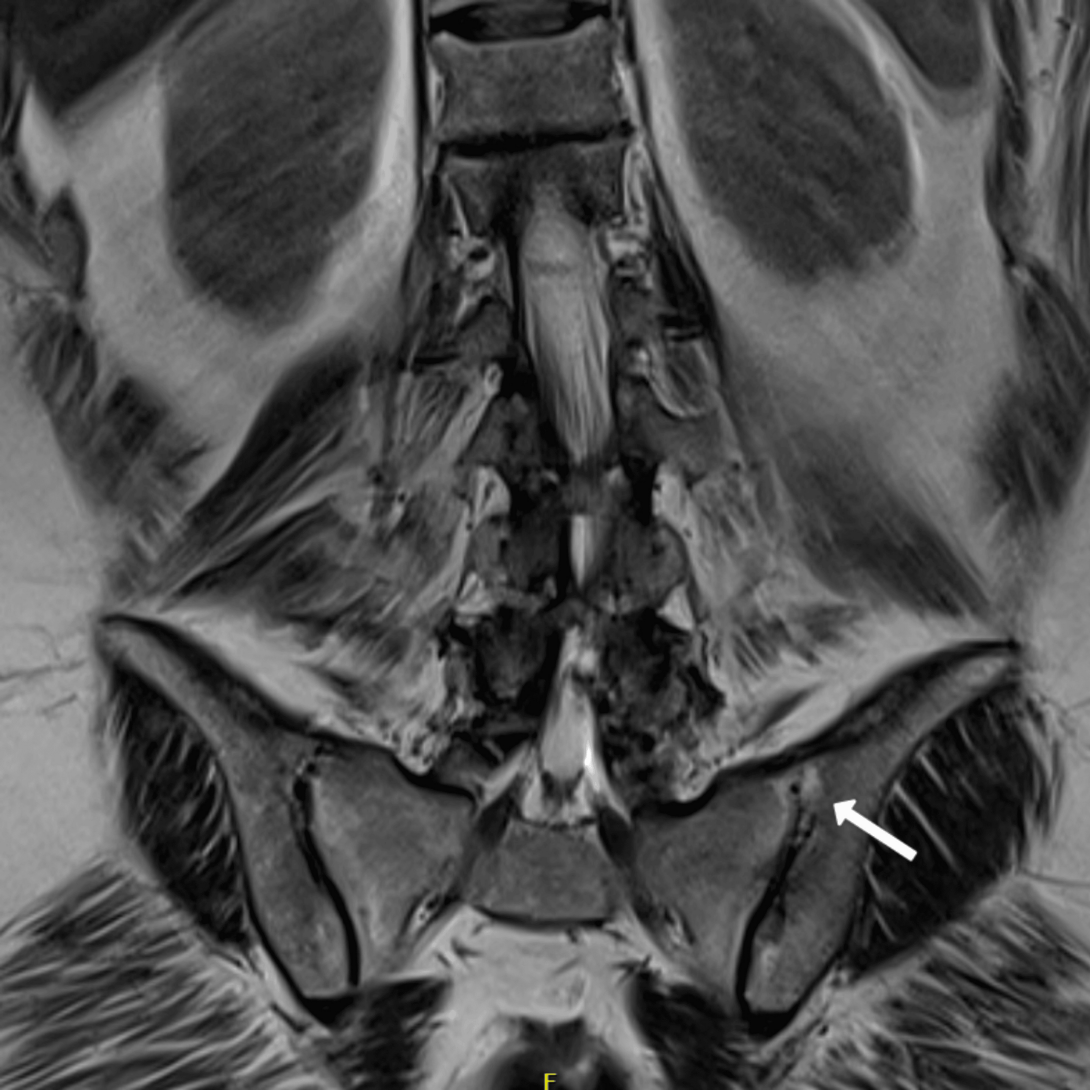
T2 fat-sensitive coronal section MRI of the sacro-iliac joints showing fatty metaplasia and ankylosis of the superficial margin of the left sacro-iliac joint (white arrow)
